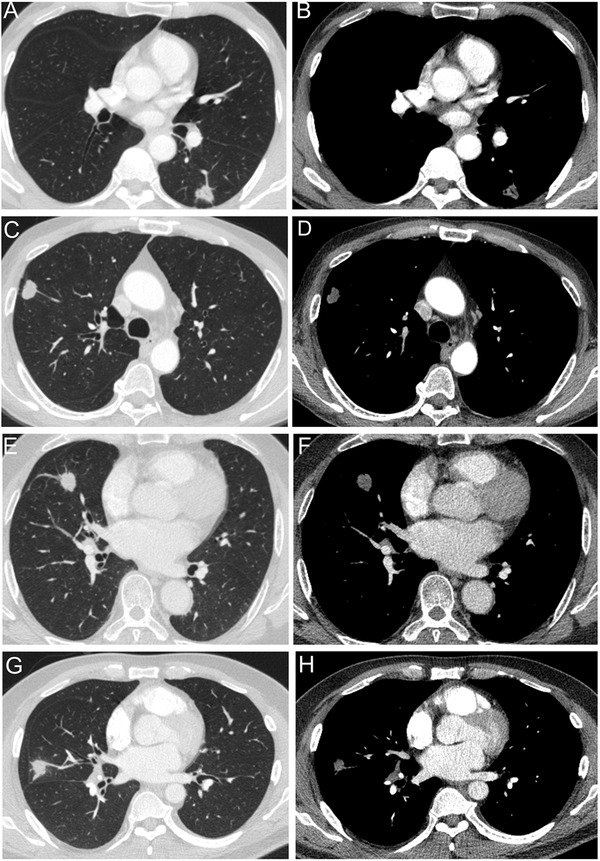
Examples of representative CT images. (A, B) Male, 65 years old, suspected malignant nodule, and pathological diagnosed as pulmonary cryptococcosis. (C, D) Male, 61 years old, long history of smoking, suspected malignant nodule and pathological diagnosed as pulmonary cryptococcosis. (E, F) Male, 57 years old, suspected pulmonary cryptococcosis, and pathological diagnosed as pulmonary cryptococcosis adenocarcinoma. (G, H) Male, 52 years old, suspected pulmonary cryptococcosis, and pathological diagnosed as pulmonary cryptococcosis adenocarcinoma
